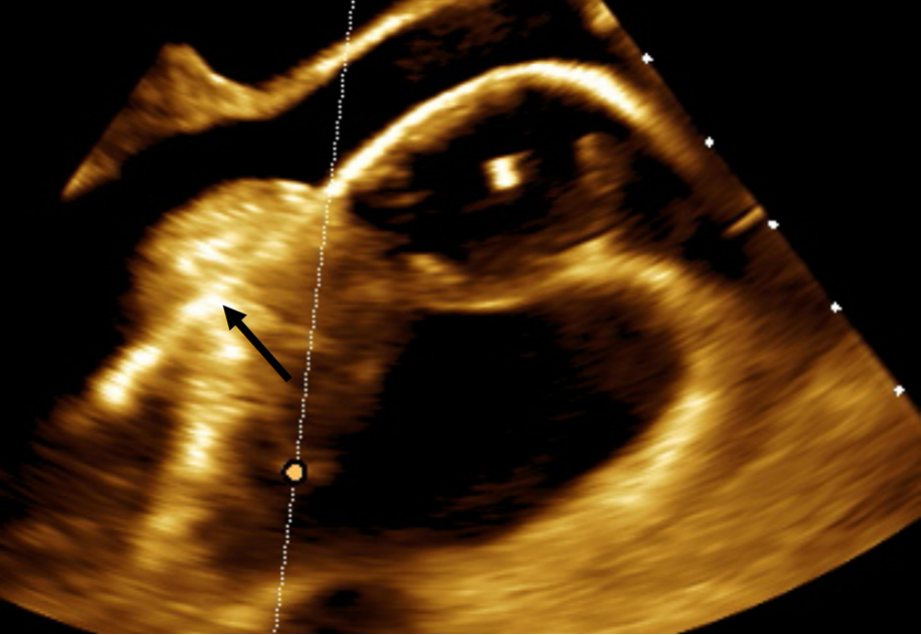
The intramyocardial course of the coaxial guidewire and microcatheter (arrow) is visualized by transesophageal echocardiography before laceration.
